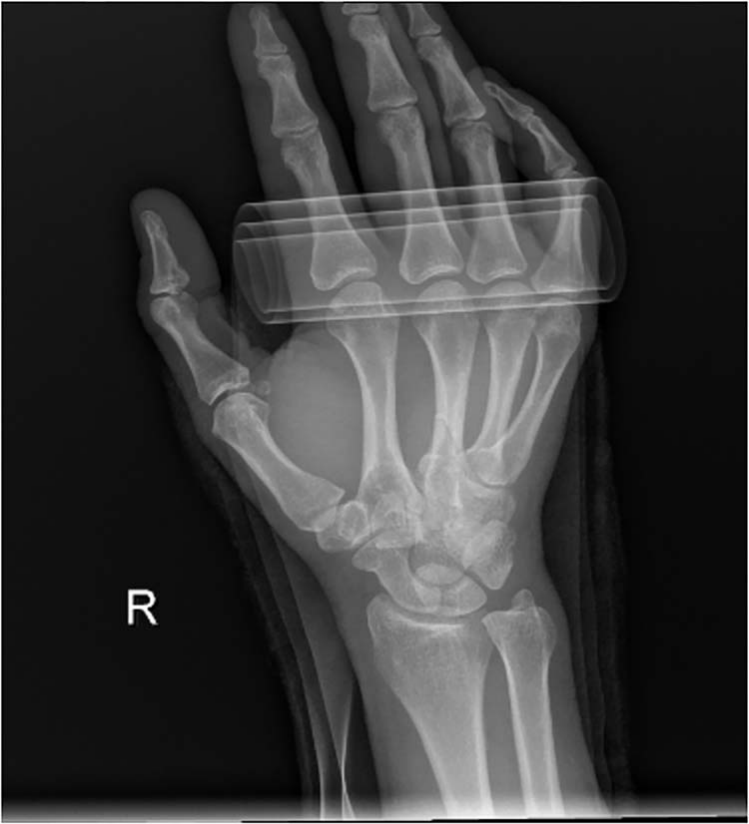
Diagnostic Radiograph 2.
